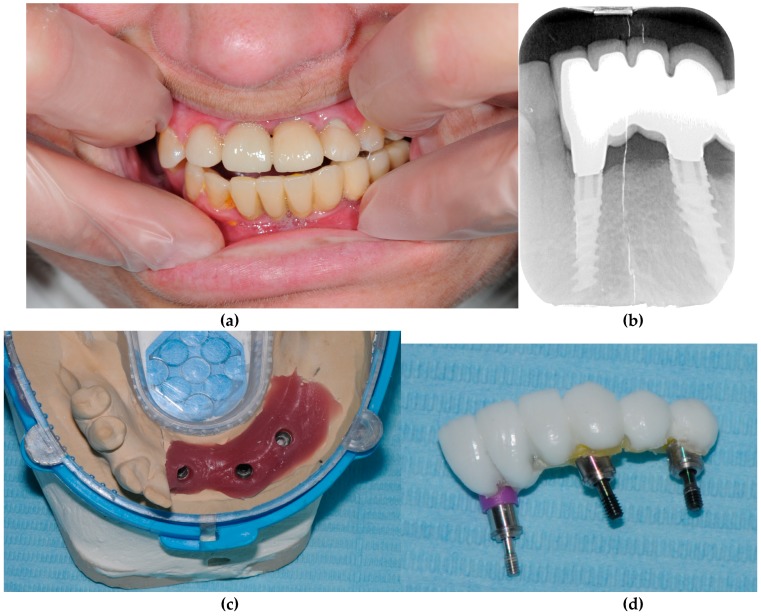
Patient 7 (-PD-FI). (a) Clinical view. (b) Radiographical view. (c) Prosthetic work model. (d) Dental prosthesis before being placed. The three implants were still in good shape two years after their placement.
